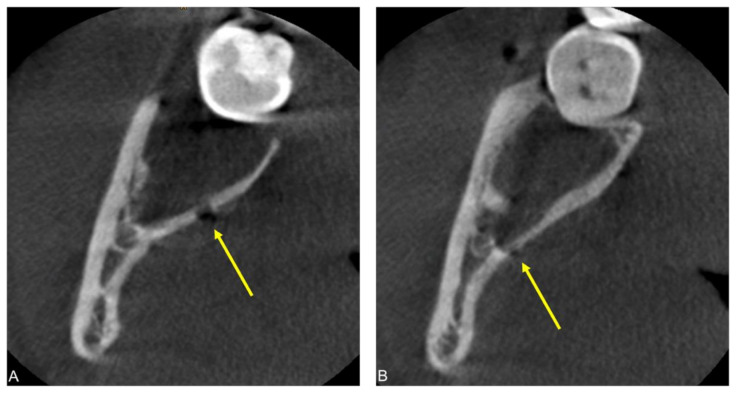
Postoperative cone-beam computed tomography (CBCT) scan of a patient complaining of persistent numbness of the right side of the tongue two weeks after bilateral MTM surgery. Axial CBCT image reconstructions (A,B) visualize two possible iatrogenic defects of the lingual cortical bone: a spherical lesion at the level of the former crown and a linear defect distal to the level of the former cementoenamel junction of the MTM.
